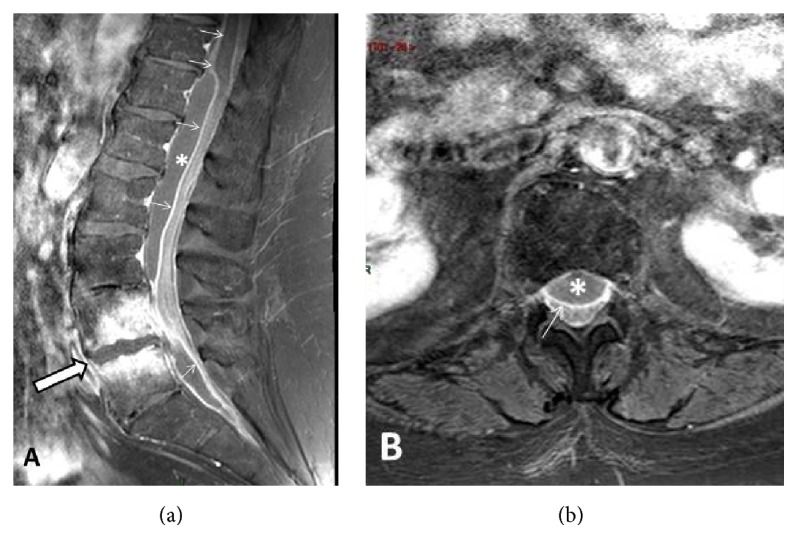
Lumbar spine MR work-up at admission. (a) Midsagittal contrast-enhanced (CE) T1-weighted view with Fat Suppression (FS) option. Spondylodiscitis at L4-L5 level (arrow) with necroticocystic intersomatic abscess and strong enhancement of the adjacent bone marrow. Epidural abscess extending from T11/T12 to S1/S2 was well seen (asterisk) together with intense enhancement of the dura (arrows). (b) Axial–transverse CE T1-weighted view through the L1 level. Anterior epidural abscess is well seen (asterisk) impinging on posteriorly displaced thecal sac. Note intense enhancement of the dura (arrow).
